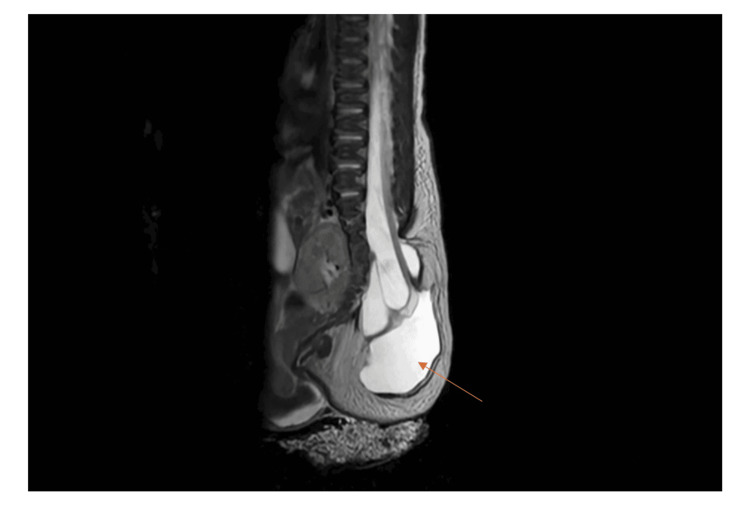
Sagittal-view T2 MRI of myelomeningocele (red arrow)
